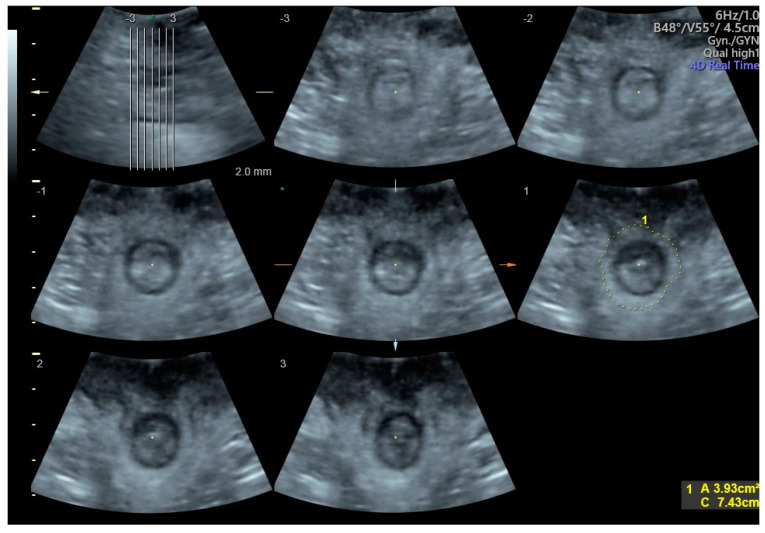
Visualization of the anal sphincter and measurement of its area using tomographic ultrasound imaging (TUI). The slices range from −3 to +3, and the green spot (*) marks the 0 point.
